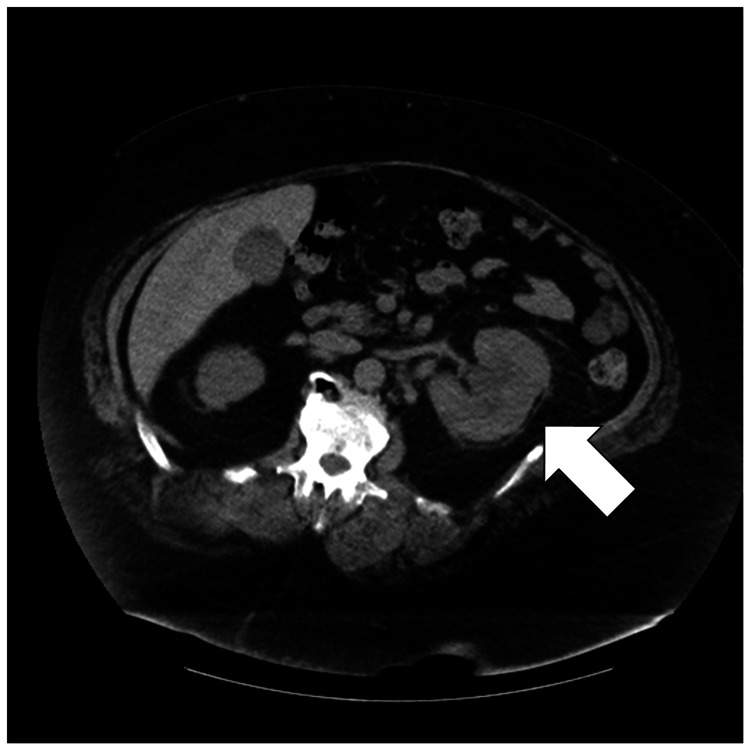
Post-discharge computed tomography (CT) imaging on day 54, performed after the patient weight had fallen below the weight limit of the CT equipment. Unenhanced CT showing a mildly enlarged left kidney and increased perirenal fatty tissue accumulation (arrow). The imaging findings were compatible with pyelonephritis, and given that the C-reactive protein levels had not normalized even after 54 days, progression to renal abscess was also considered probable. However, a definitive diagnosis could not be reached based on non-contrast CT imaging findings.
